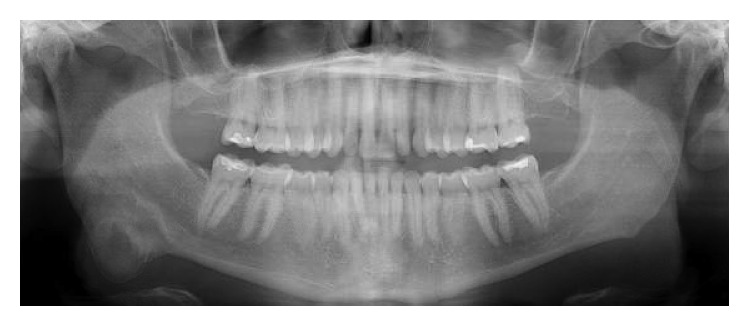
Preoperative orthopantomogram revealing a mixed (radiolucent-radiopaque) lesion, the inferior border of the mandible in the right angle region.
